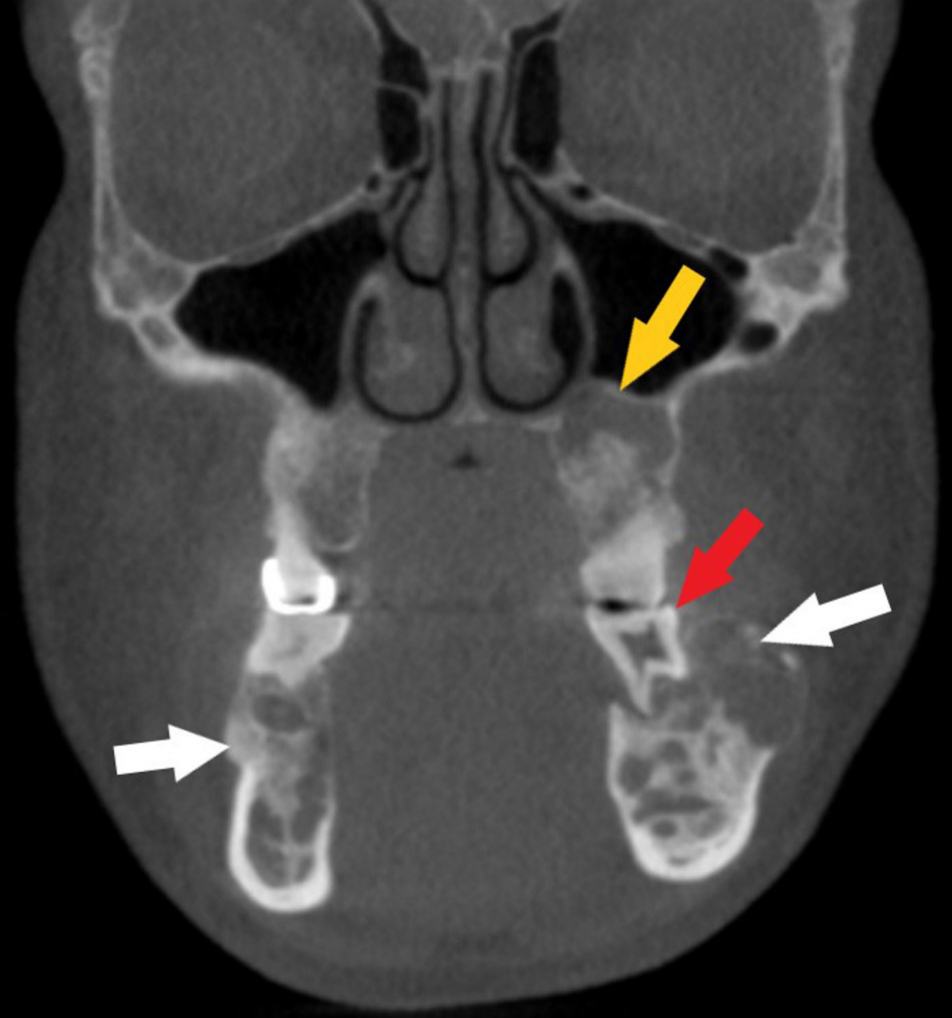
Coronally reconstructed CBCT of the jaws depicting a mixed lytic trabecular pattern and displacement of buccal cortical plate (white arrows). Taurodontism with shortened roots noted in #19 (red arrow). Superiorly displaced floor of the left maxillary sinus (yellow arrow). CBCT, cone beam CT.
